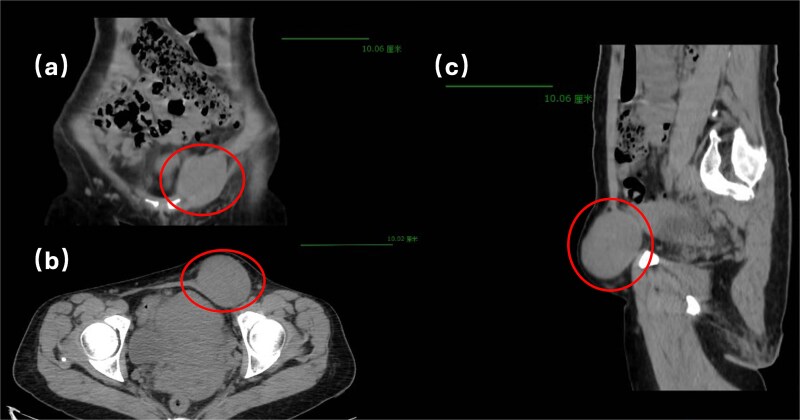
Pelvic computed tomography.
